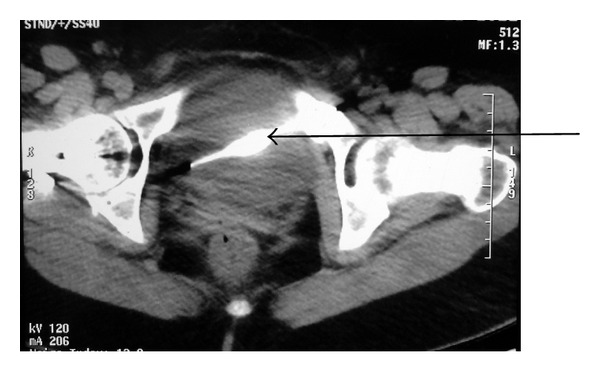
Computed tomography showing K-wire lying in the soft tissues of the pelvis.
